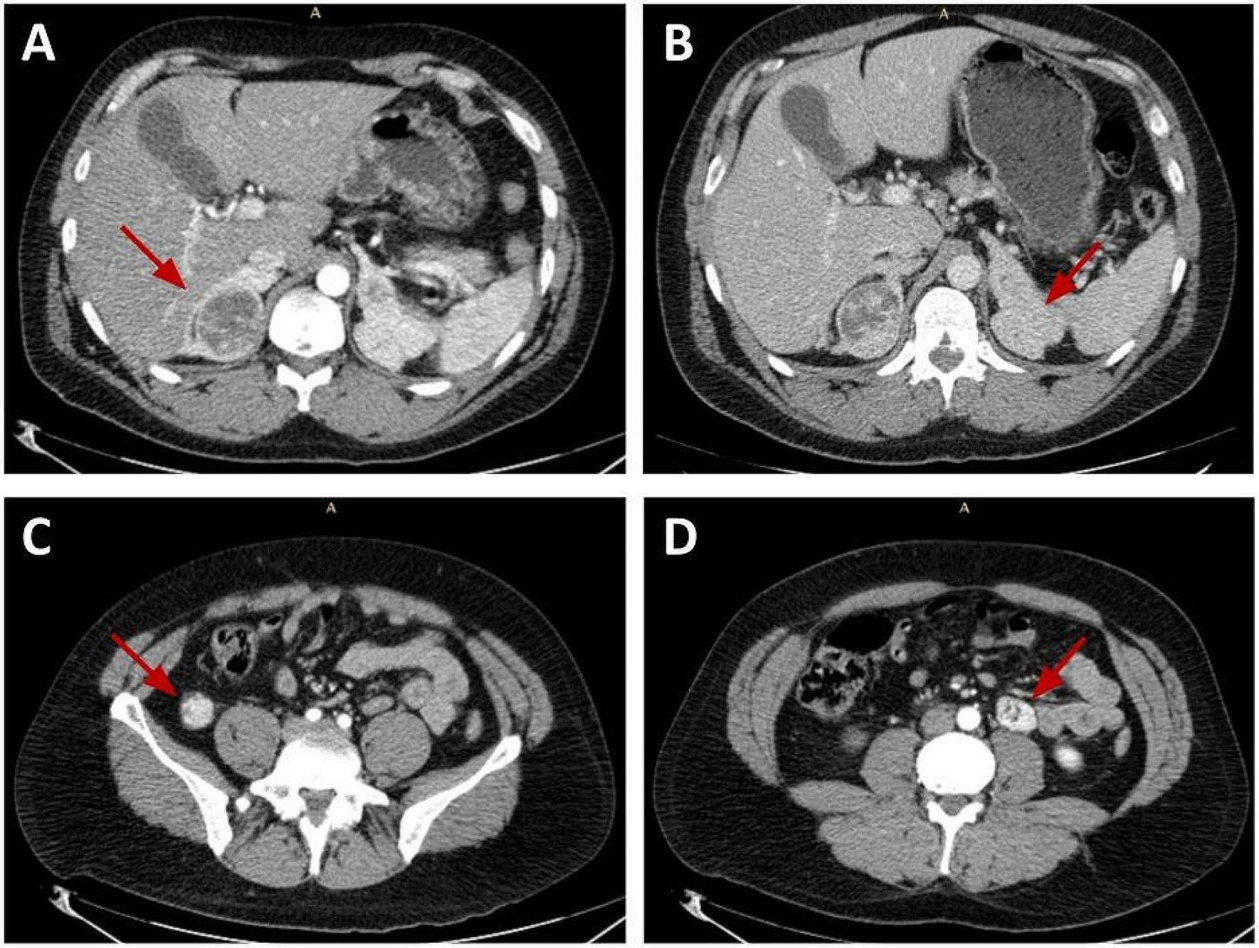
Contrast enhanced CT scan: (A) Right adrenal gland lesion of 5 cm with a central colliquation area; (B) major left adrenal gland lesions of 4,2 cm; (C) paralumbar right lesion of 2.6 cm; (D) lesion in left peri-cecal fat of 3.1 cm
